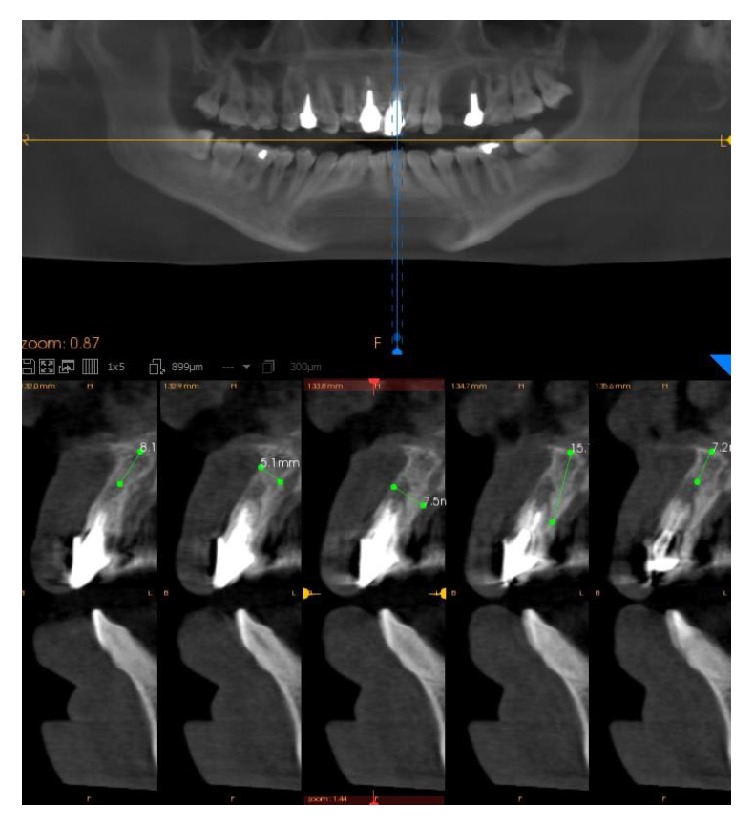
Cone beam computed tomography (CBCT). The cone beam computed tomography (CBCT) examination confirms the presence of the tooth resorption. A careful 3D analysis of the height and width of the alveolar ridge is performed, in order to better plan the implant placement.
